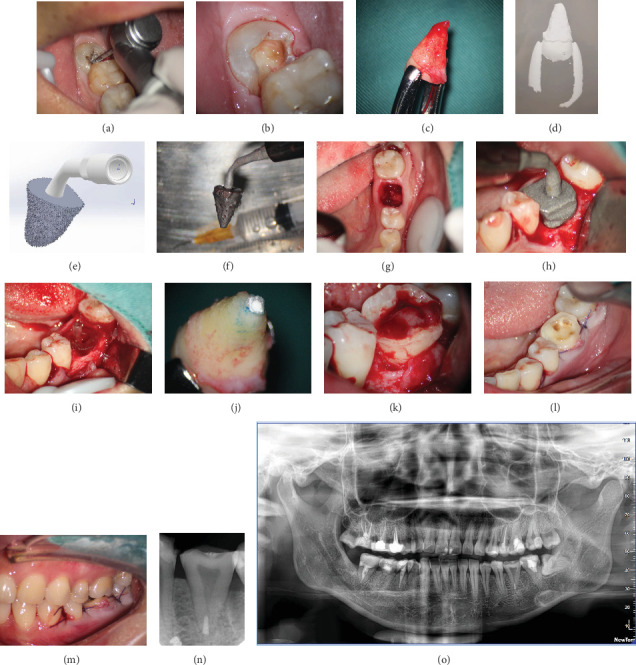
The clinical procedure. (a) Removal of dental caries on the mesial–occlusal part of Tooth #32 using a fissure bur. (b) Tooth #32 after removal of carious tissue. (c) Tooth #32 was extracted with a minimally invasive technique. (d) Tooth #32 was scanned digitally by a 3Shape scanner to obtain a highly accurate 3D image. (e) A custom 3D ultrasonic osteotome was designed. (f) A 3D-printed ultrasonic osteotome. (g) Occlusal view of recipient alveolar socket. (h) A 3D-printed individual ultrasonic osteotome was used to prepare the recipient's alveolar socket. (i) A CARP model was used to evaluate and prepare the receptor alveolus. (j) The root end of Tooth #32 was prepared and filled with iRoot BP. (k) The prepared Tooth #32 was transplanted into the recipient site. (l) Occlusal view of the transplanted tooth with suture 1 week after surgery. (m) Buccal view of the transplanted tooth with suture 1 week after surgery. (n) Periapical radiograph of the transplanted tooth 1 week after surgery. (o) Panoramic radiograph of the transplanted tooth 1 week after surgery.
